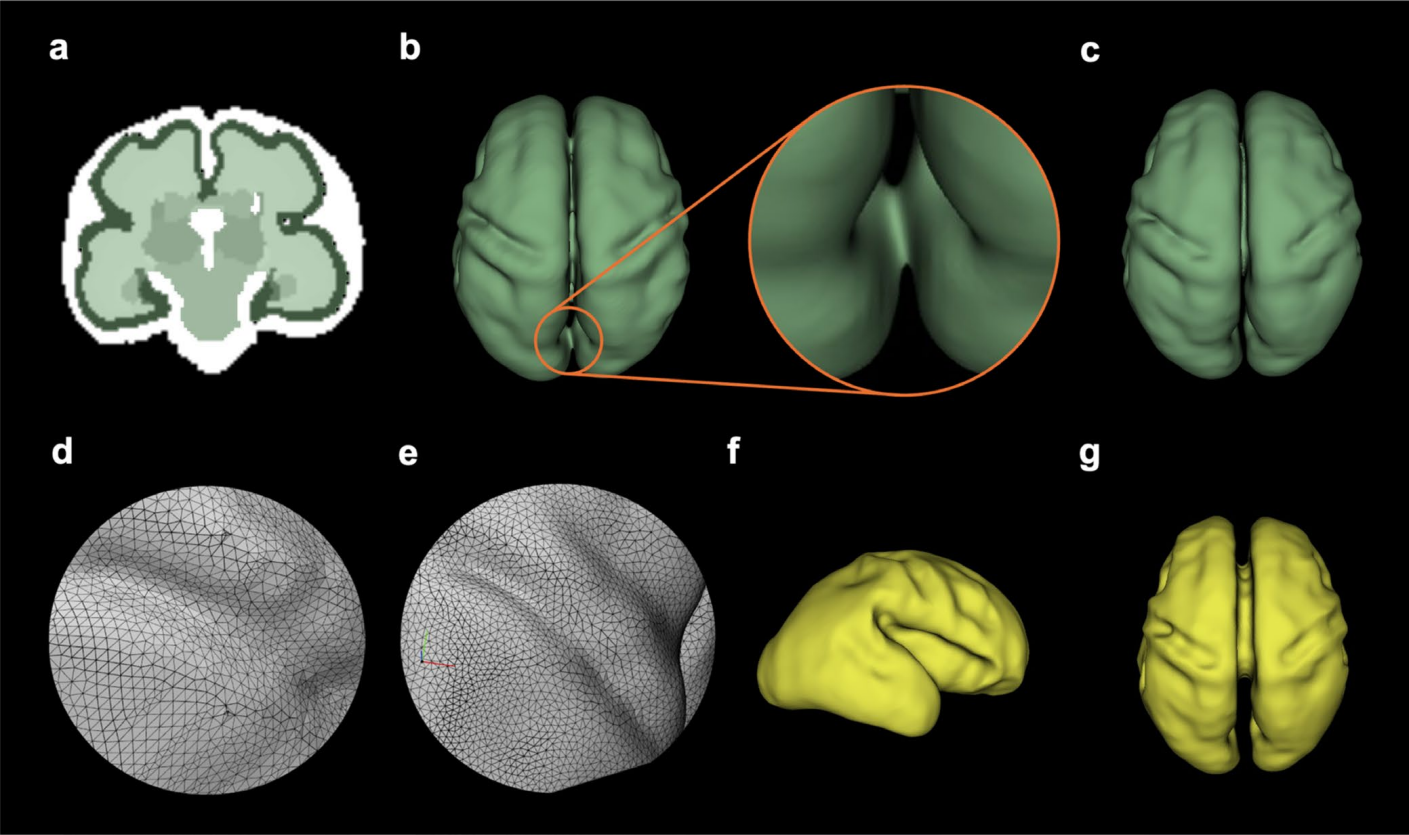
Reconstruction of pial and white matter surfaces. (a) MRI image of a case at 27 gestational weeks with automatic tissue segmentations overlaid on the anatomy (in this study we only used the white matter‐cortical gray matter surfaces and did not use the other segmented structures). (b) Pial surface from automatic gray matter segmentation without manual modification, showing a close‐up of a structural error. (c) Pial surface after manual modification. (d) Irregular mesh of the surface. (e) Surface after smoothing and uniform remeshing, (f–g) Sagittal and axial views of the white matter surface.
